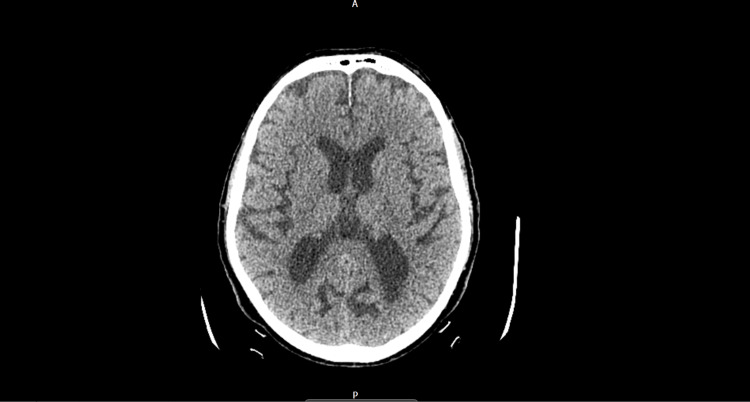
Non-contrast CT head. Non-contrast CT head of the patient done during the admission did not reveal any acute intracranial abnormalities/stroke or bleeding. CT, computed tomography
